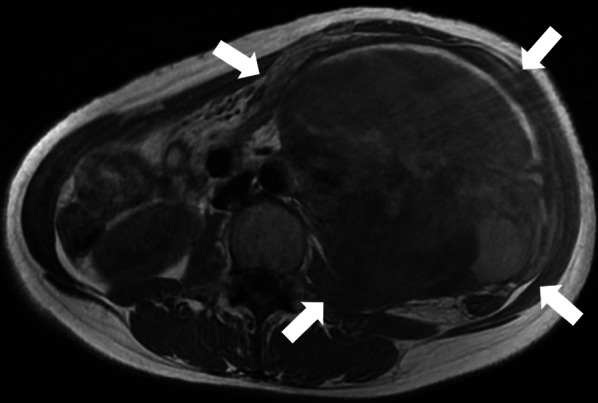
The tumor, with a maximum size of 13 × 13 cm, is located in the left lateral abdomen (arrow). The tumor is uniformly high-intensity with partial low intensity on T2-weighted image
